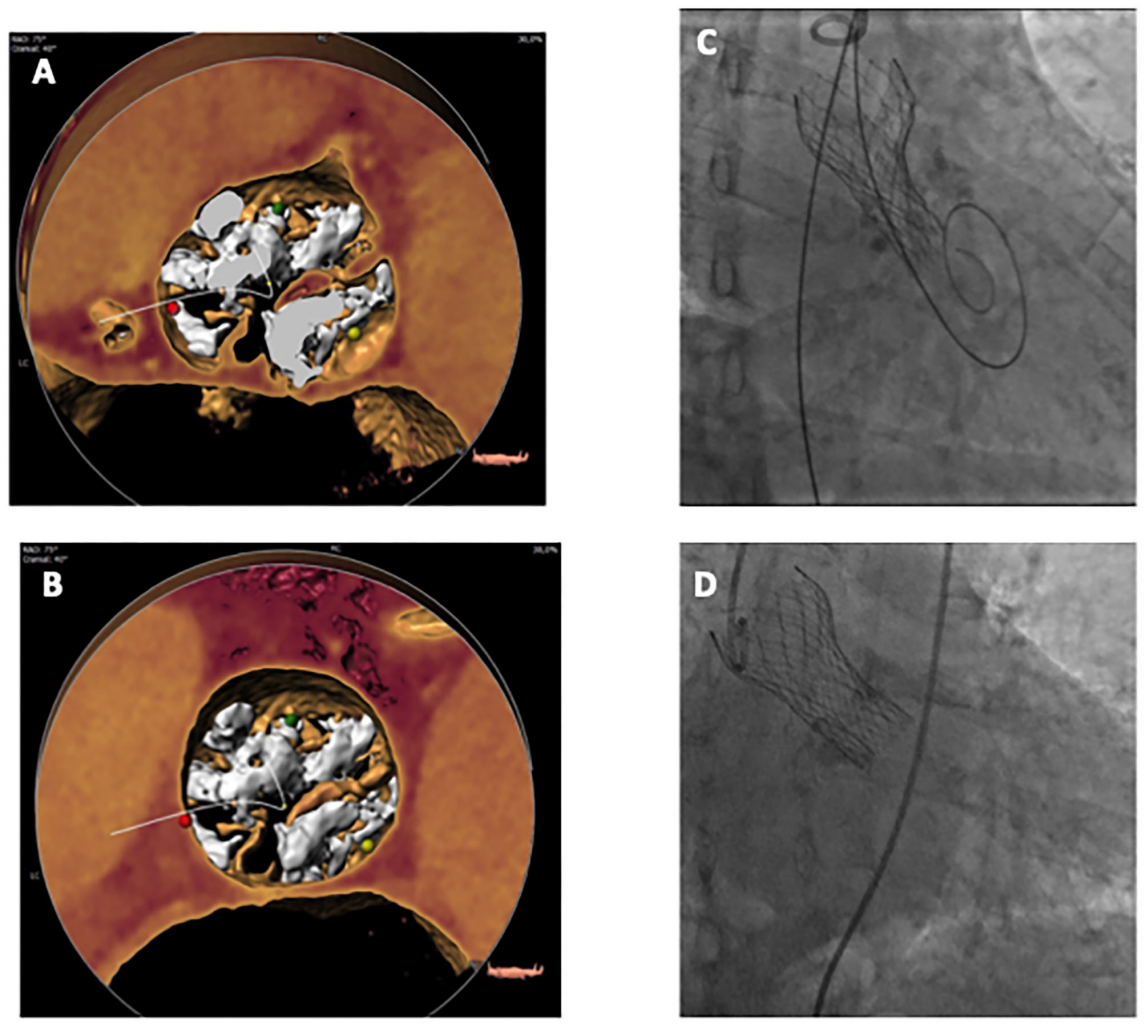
3D Volume Rendering MSCT view of a BAV at annulus level (A) and raphe level (B), in this case at 10.4 mm from annulus plane. Constrained THV right after release (C) and final result after post-dilatation (D). BAV, bicuspid aortic valve; MSCT, multislice computed tomography; THV, transcatheter heart valve.
